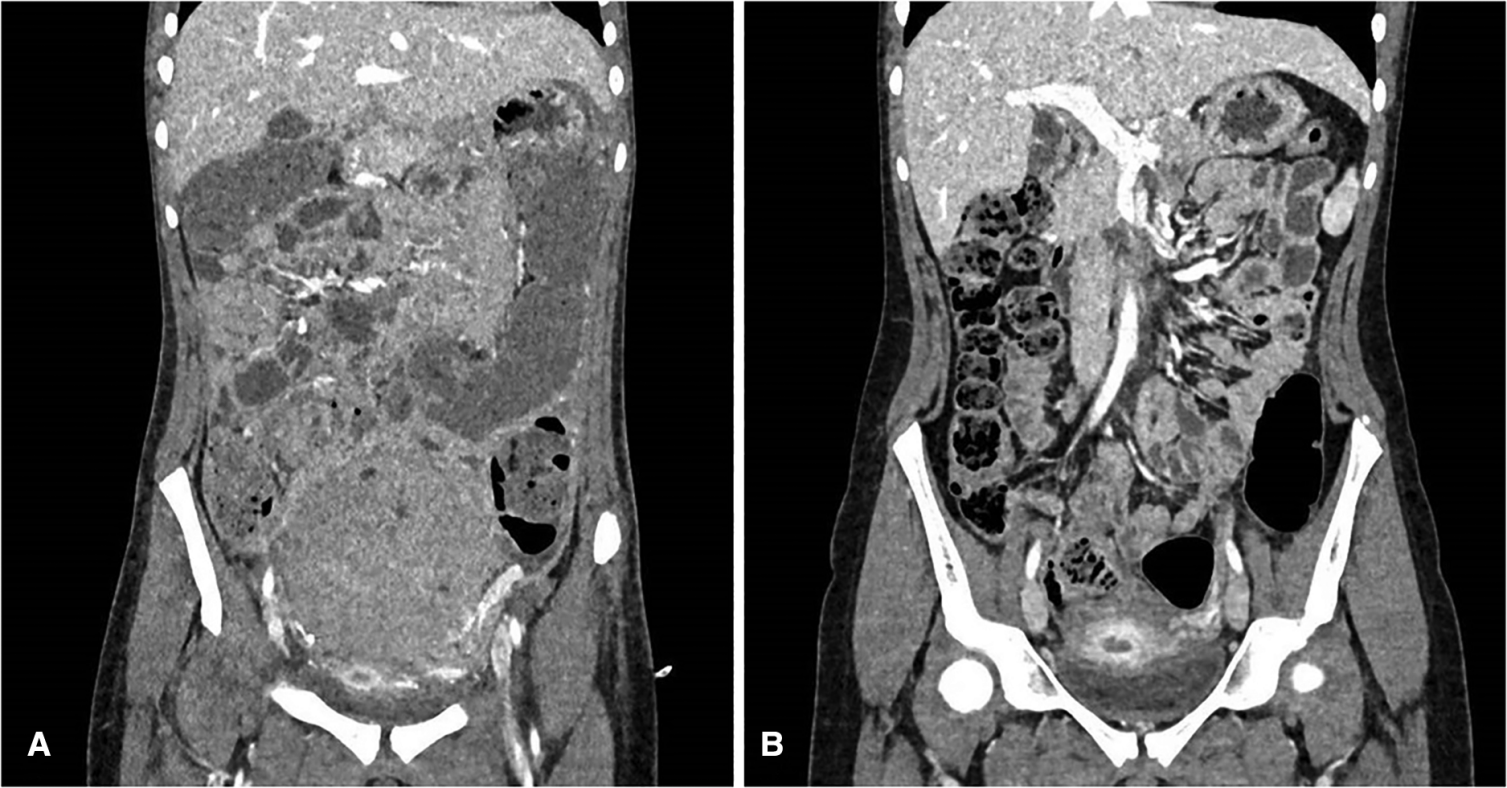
(A) CT scan after surgery (before chemotherapy); (B) CT scan after four cycles of chemotherapy.
